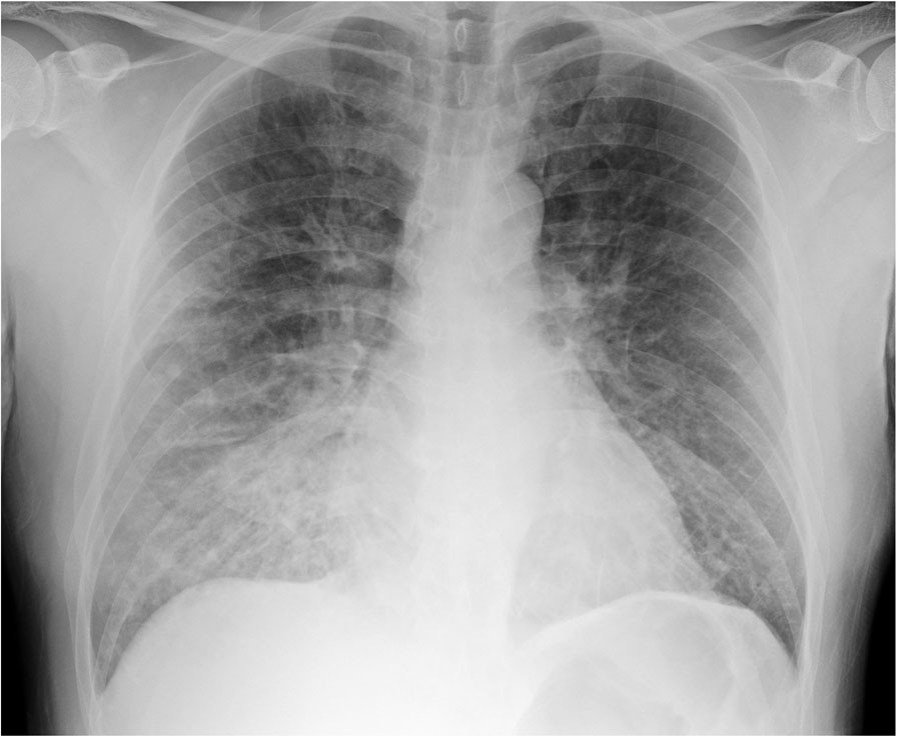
Chest X-ray revealing indistinct pulmonary vessels, diffuse opacities in the lower lung zones, which were more prominent in the right lung
